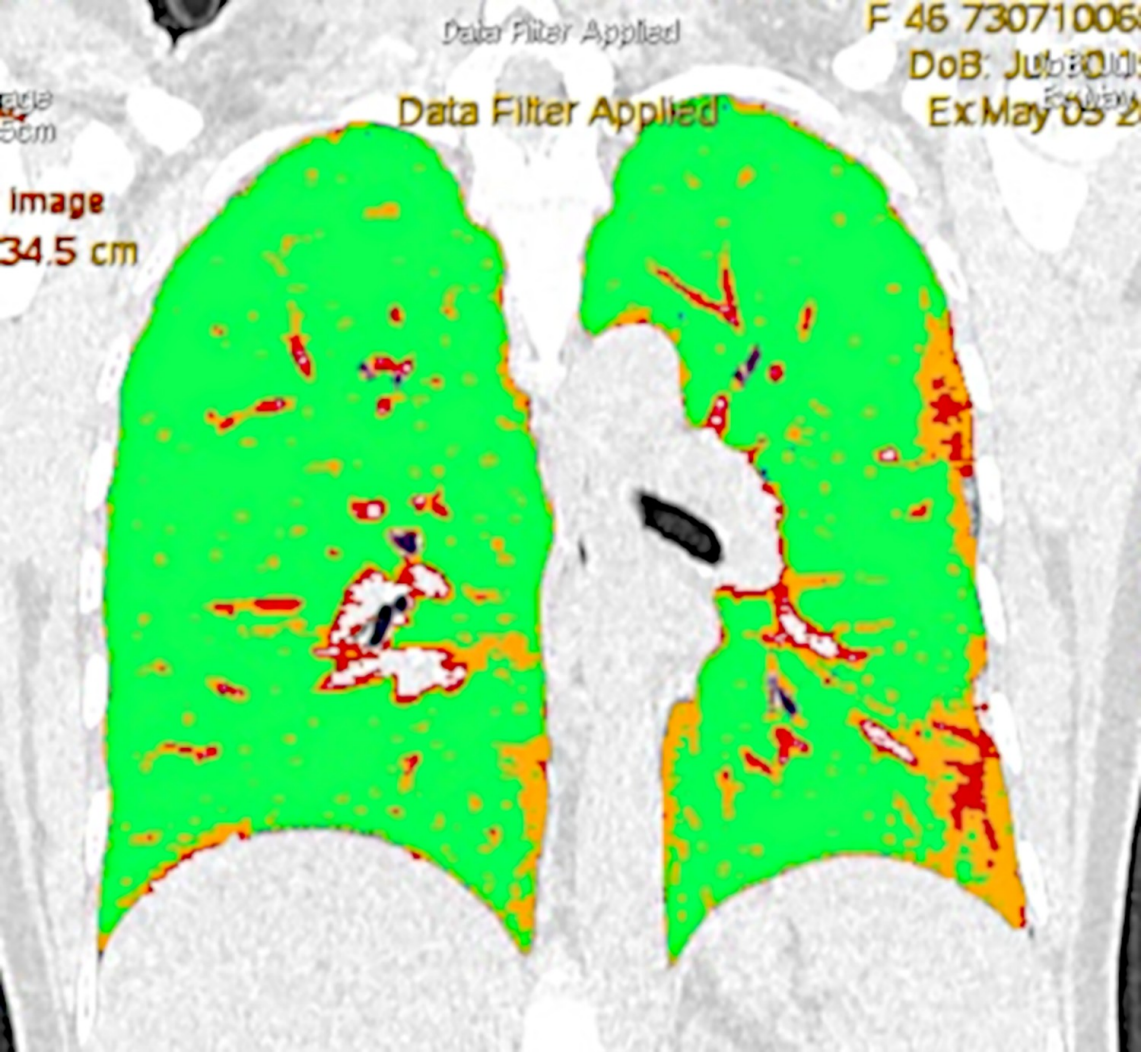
Quantitative computed tomography (QCT) with Thoracic VCAR software in the non-severely ill patient: Orange color—ground-glass opacities 12,2597% total lung volume involved; red color—consolidation 6,2129% total lung volume involved); blue color–emphysema 0,0940% total lung volume involved; green color—normal parenchyma 81,4334% total lung volume.
